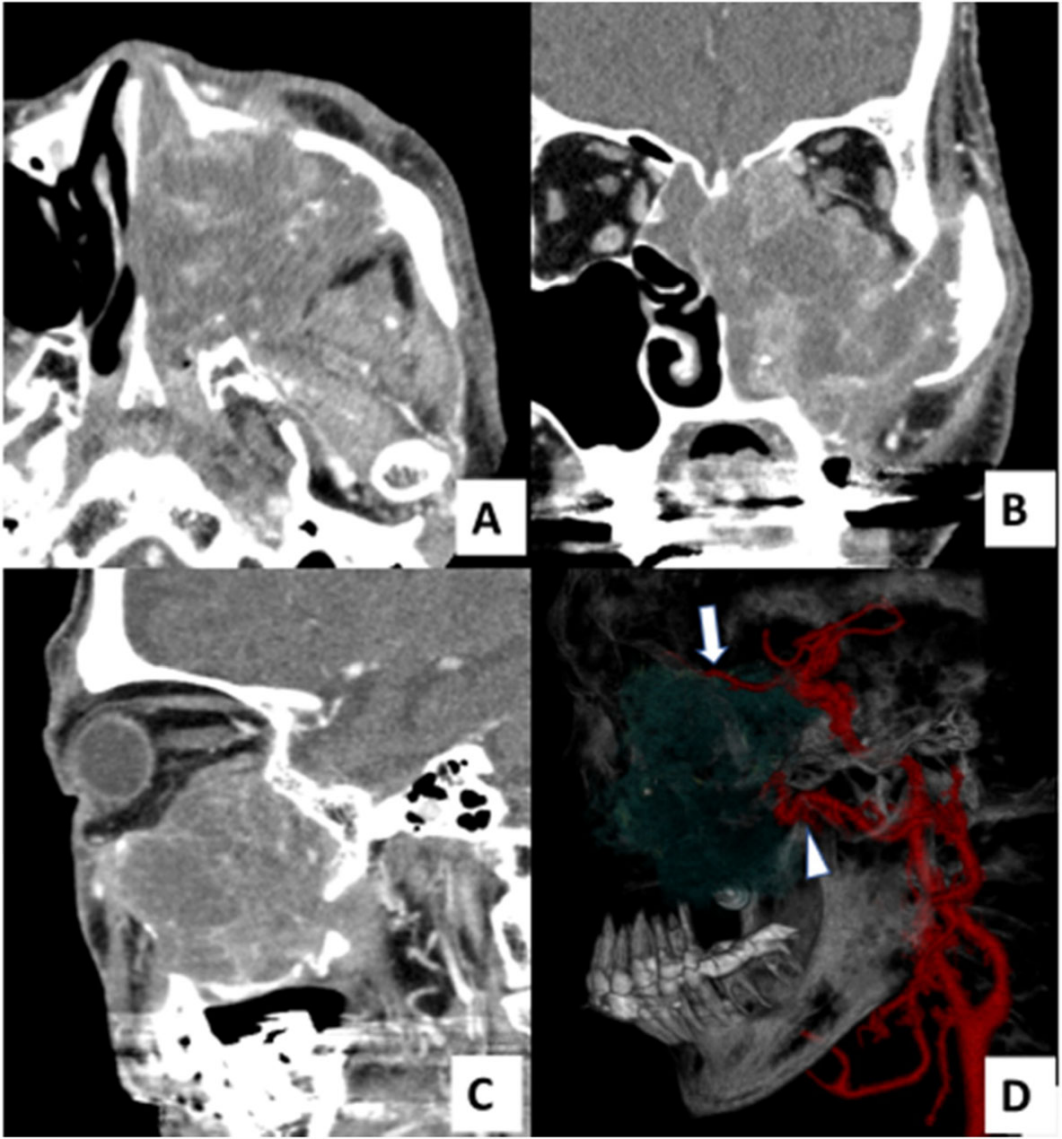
Imaging of recurrent left maxillary carcinoma. A head and neck contrast-enhanced computed tomography showed an irregularly demarcated mass with orbital invasion. a Axial image; b Coronal image; c Sagittal image. d Feeding artery was suspected to involve the ophthalmic artery (white arrow) as well as the maxillary artery (white arrowhead)
